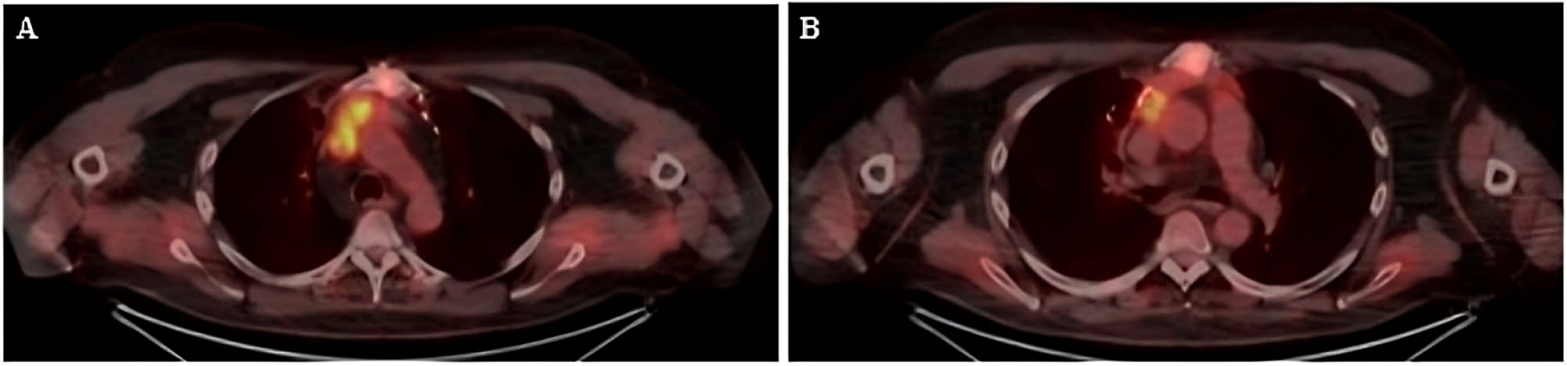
CT-PET axial sections showing avidity of the mass throughout the mediastinum (A). Avidity of the tail of the mass below the subcarina (B)
